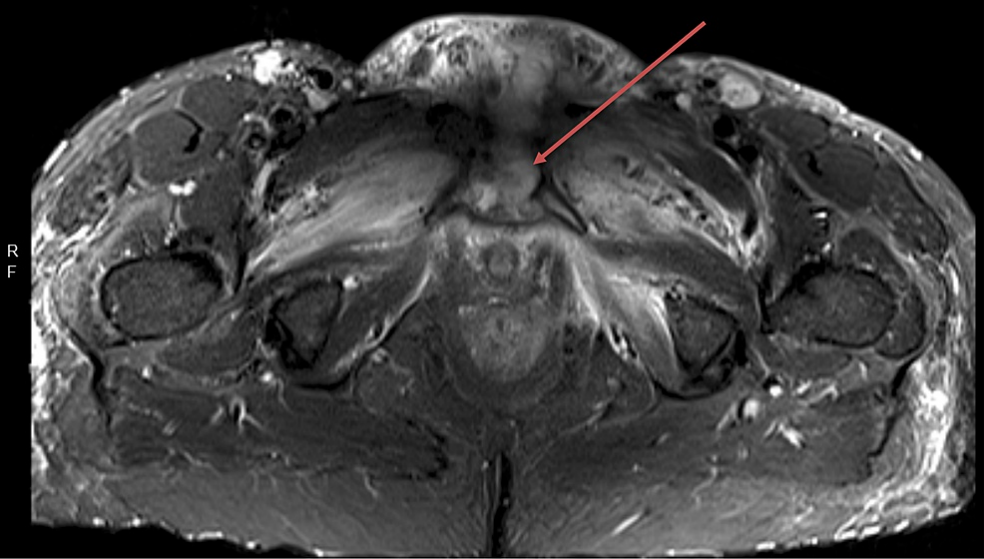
Short-T1 inversion recovery (STIR) MRI of the pelvis. Red arrow indicates destructive changes of the symphysis pubis with osteomyelitis of the pubic bodies.
